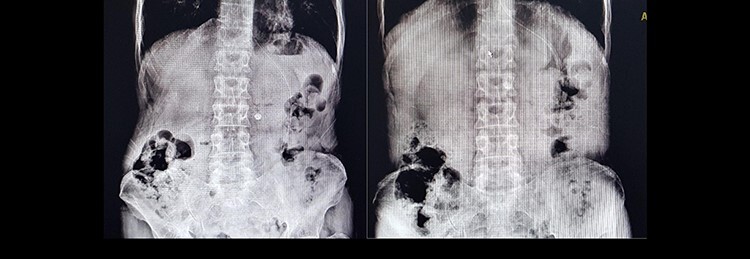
Supine and upright abdominal X-ray.
